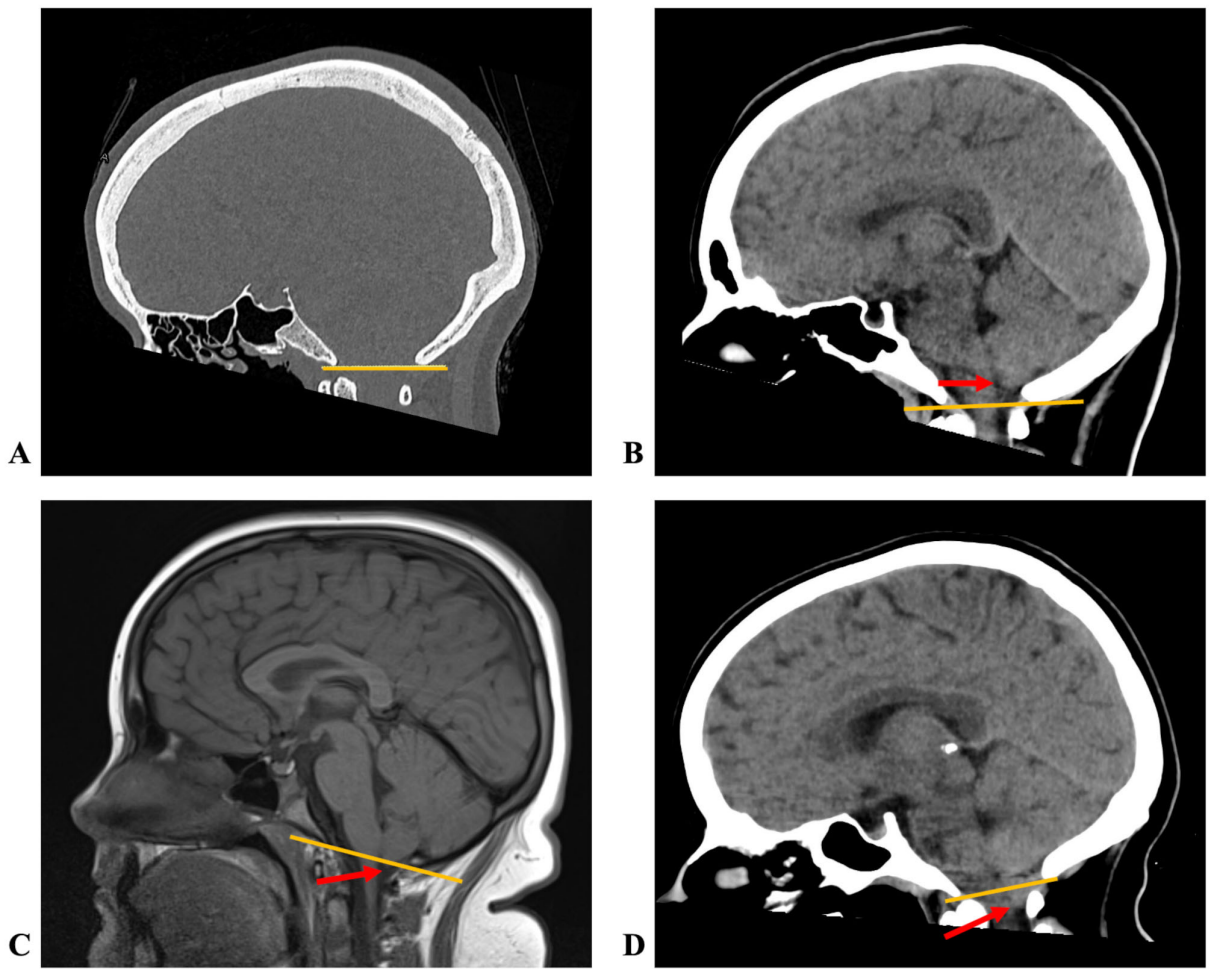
Sagittal images showing the McRae line in yellow; red arrows indicate the tip of the cerebellar tonsil. (A) CT bone window; (B) CT with the cerebellar tonsil above the line; (C) T1-weighted MRI with the cerebellar tonsil at the line; (D) CT with cerebellar tonsillar ectopia.
